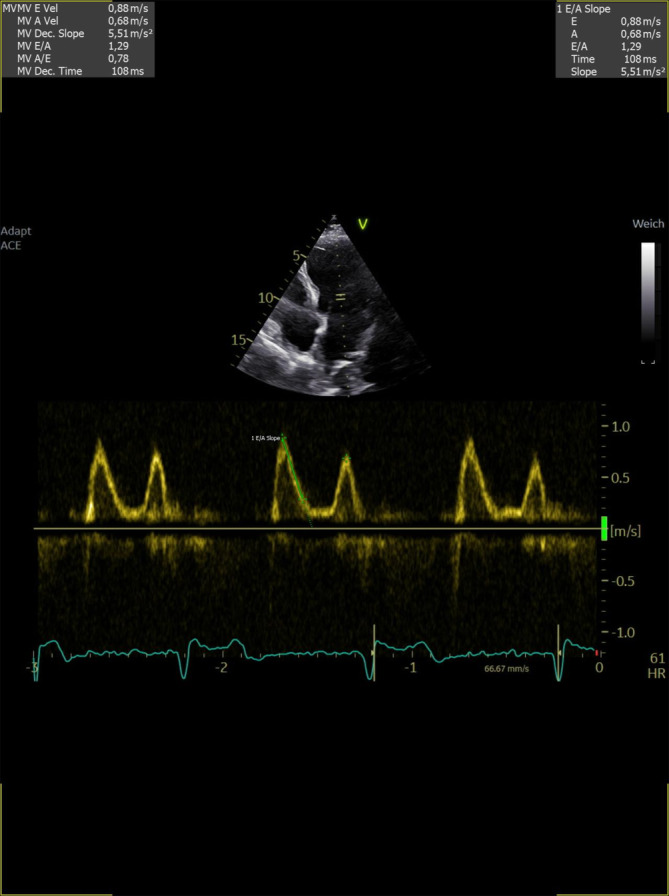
Bestimmung der diastolischen Funktion mittels Pulsed-wave-Doppler des Mitraleinstromsignals. Hier liegt ein pseudonormales Muster mit verkürzter Dezelerationszeit (108 ms) vor
